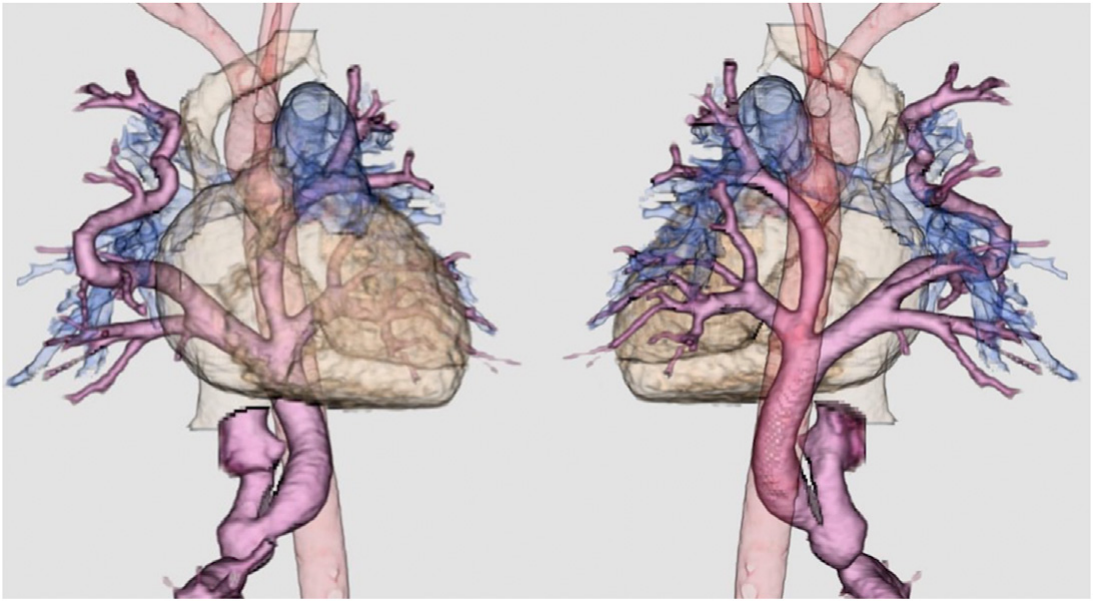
Computed tomography findings. The common pulmonary vein (PV) is hypoplastic. The right PV merges into 1 PV in the lung and forms a long branch, and the left PV has upper and lower portions, which become a short common branch behind the inferior vena cava–right atrium junction.
